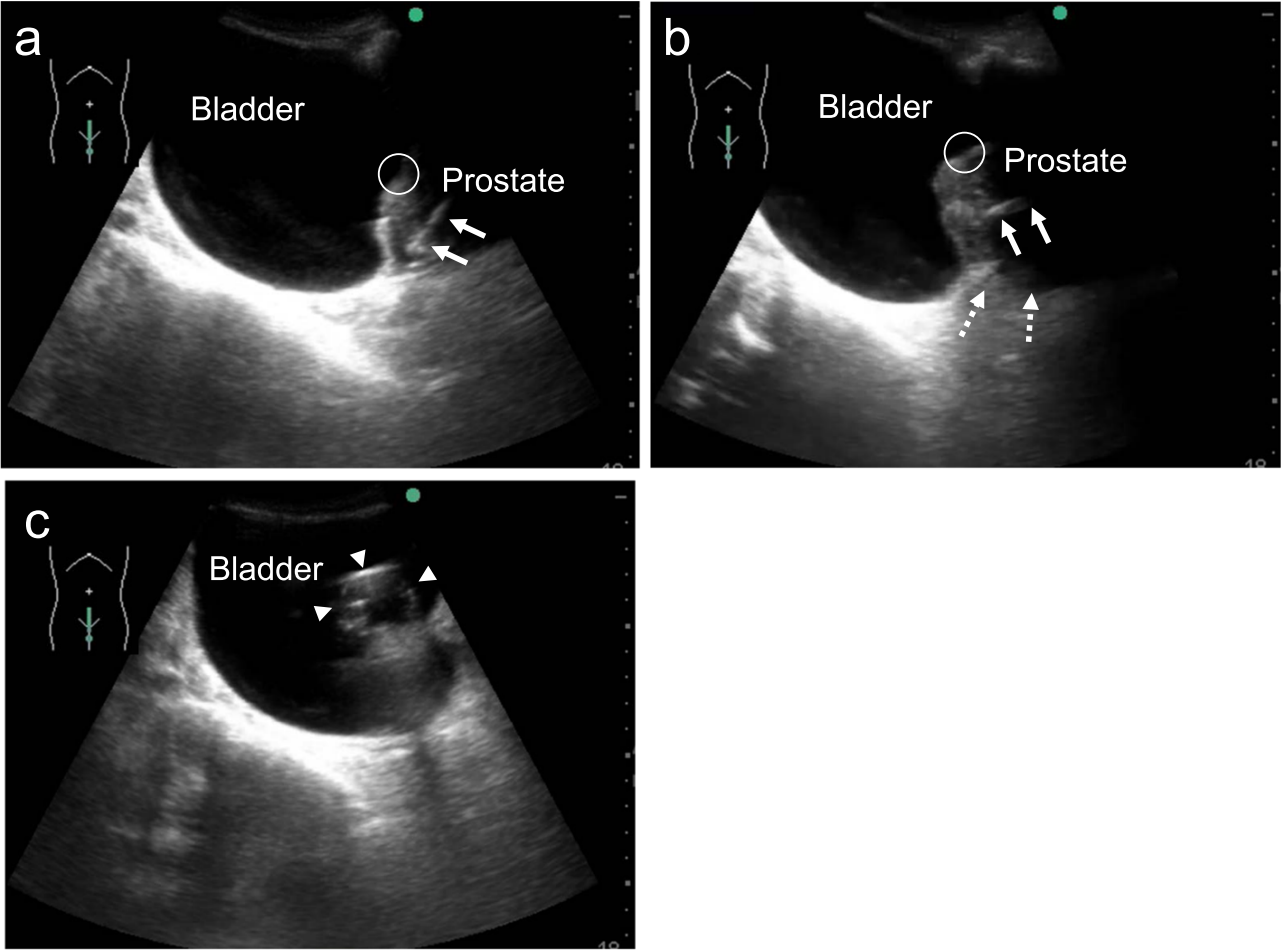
Ultrasound images in a 78-year-old man who presented with difficult urination. The patient had a history of benign prostatic hypertrophy. Standard urethral catheterization attempted by an experienced emergency nurse and an experienced emergency physician failed due to complicated urethral bleeding. a Bedside ultrasound revealed the tip of the catheter in a part of the posterior and bulbar urethra (*arrows*) while the progress was obstructed. Judging from the location of the internal urethral orifice, a part of the urethra was thus determined to be bent. The *circle* denotes the location of the internal urethral orifice. b The bent part of the urethra had become blunt with transrectal pressure using an inserted index finger (*broken arrows*). The *arrows* denote the tip of the catheter, and the *circle* denotes the location of the internal urethral orifice. c Ultrasound-guided catheterization with transrectal pressure without forceful manipulation was successful on the first attempt. *Arrowheads* denote the inflated balloon
